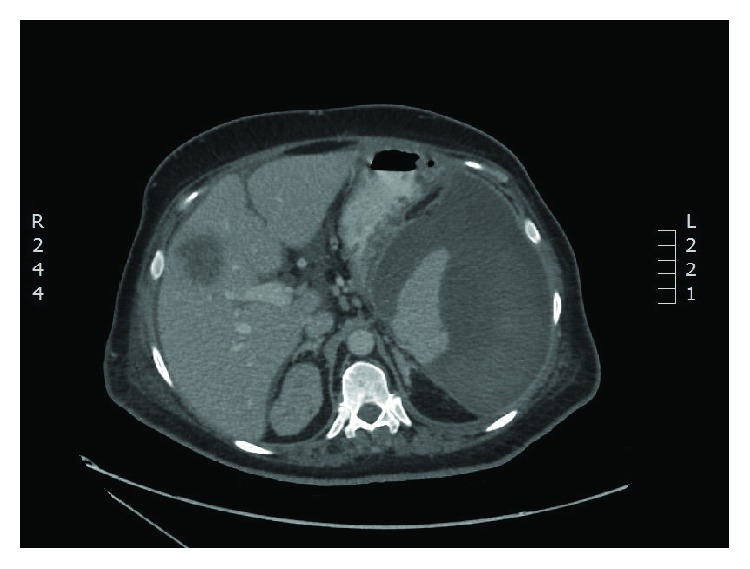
CT scan of the abdomen with IV contrast showing a large subcapsular splenic hematoma with gas formation.
